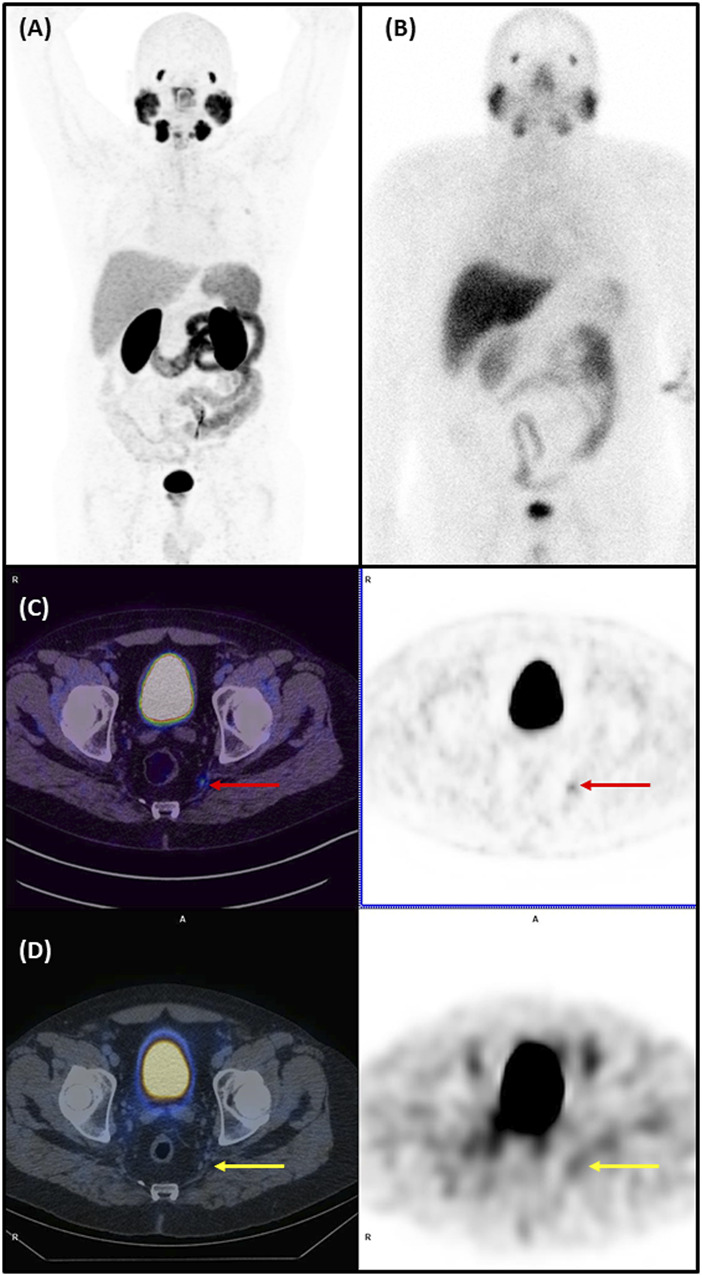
[^68^Ga]Ga-PSMA-11 PET/CT and [^99m^Tc]Tc-PSMA-I&S SPECT/CT of a 59-year-old man with a Gleason score of 7 (4 + 3) prostate cancer (PCa). (A) The maximum intensity projection (MIP) of [^68^Ga]Ga-PSMA-11 PET/CT imaging (anterior view) performed for staging. (B) [^99m^Tc]Tc-PSMA-I&S whole body scan (anterior view), obtained the day before radioguided surgery. (C) [^68^Ga]Ga-PSMA-11 PET/CT of the pelvic region: *Left* - fused PET/CT axial slice of the pelvis showing abnormal uptake in a small (8 mm) left internal iliac lymph node (red arrow); *Right* - corresponding PET axial slice, pinpointing abnormal [^68^Ga]Ga-PSMA-11 uptake in the above mentioned lymph node. (D) [^99m^Tc]Tc-PSMA-I&S SPECT/CT of the pelvic region: *Left*–fused SPECT/CT axial slice of the pelvis showing slight abnormal uptake in the same small (8 mm) left internal iliac lymph node (yellow arrow); *Right* - corresponding SPECT axial slice, pinpointing abnormal [^99m^Tc]Tc-PSMA-I&S uptake in the earlier quoted lymph node. _
